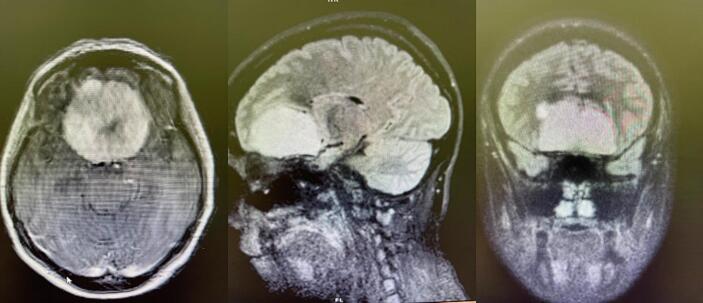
Brain computed tomography with contrast demonstrates a large subfrontal tumor.
